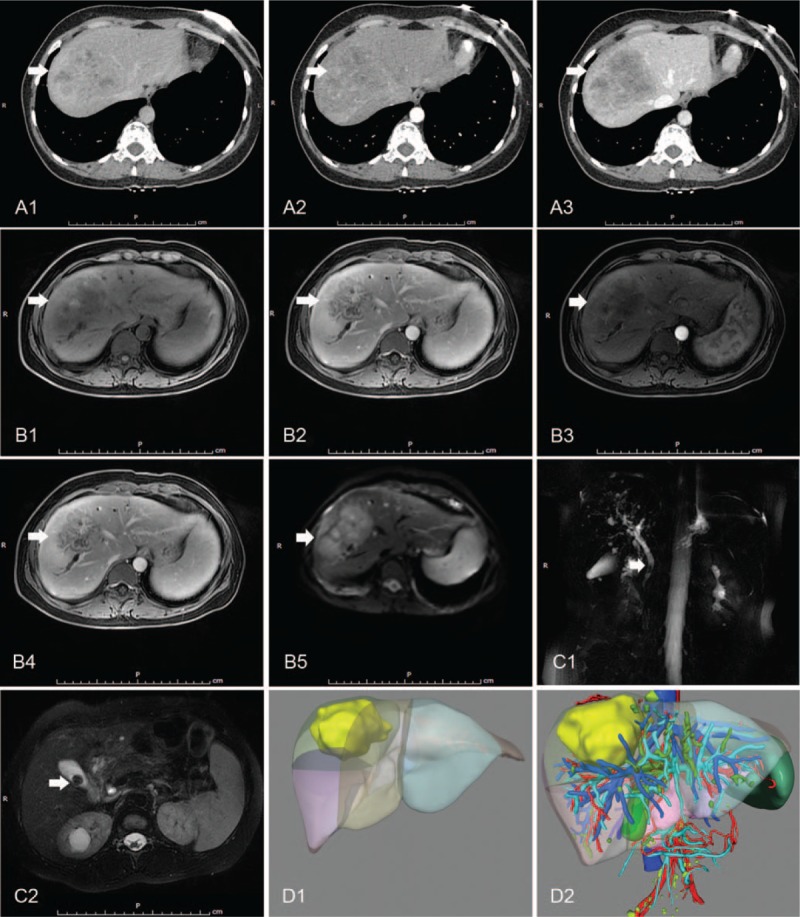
Preoperative radiological examination of the reported case. (A, B) Abdominal computed tomography (CT) scanning and abdominal MRI scanning showed a hypoattenuating liver lesion which located in the segment 8 and was adjacent to right and middle hepatic vein. From (A) to (B), the white arrow heads direct the liver lesion. (C) Magnetic resonance cholangiopancreatography (MRCP) scanning showed calculi were present in the main, left hepatic bile ducts and cholecyst; the white arrow heads direct the calculi. (D) 3D reconstruction of CT images. The liver lesion is labeled with yellow, hepatic artery are labeled with red, portal vein and its branches are labeled with cyan, and hepatic vein and inferior vena cava are labeled with blue.
